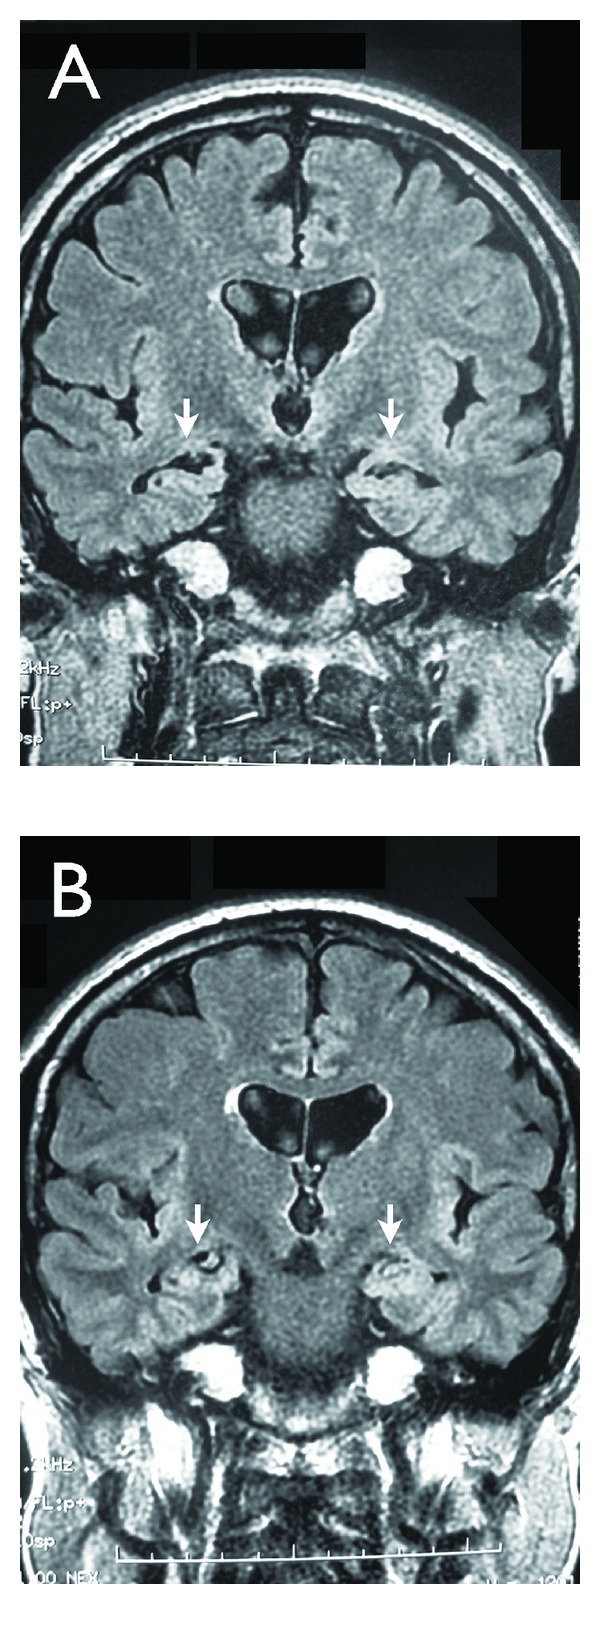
Fluid-attenuated inversion recovery MRI images of the brain in Case 2. MRIs were performed (A) in December 2008 and (B) November 2011. Arrows indicate the hippocampus.
